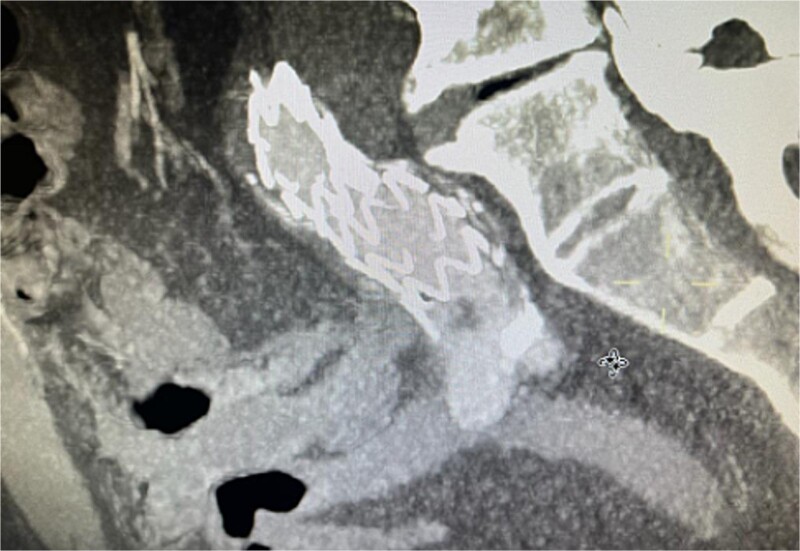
CTA scan showing the floating thrombus distal to the right iliac artery stent, following the patient presenting with acute embolic ischemia in the right leg.
